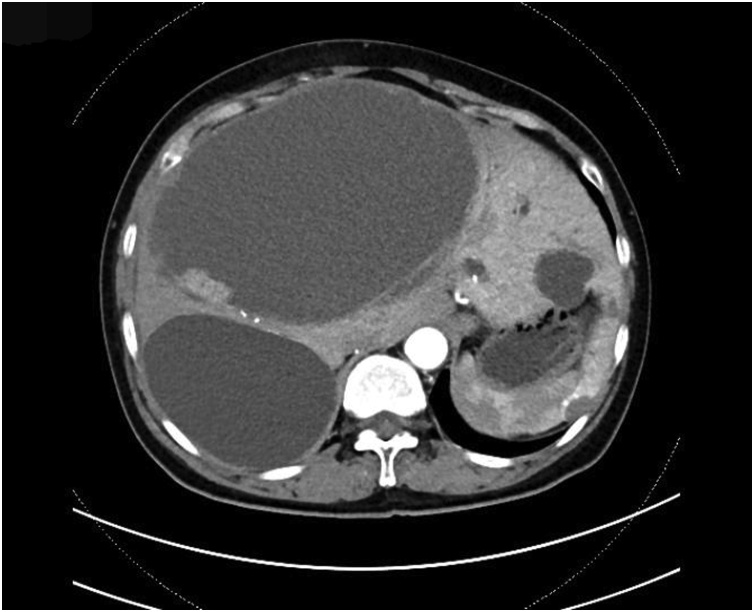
CT scan showing hepatic multiple cysts.
